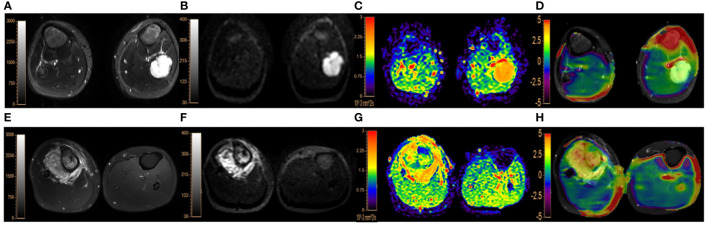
(A–D). A 9-year-old male patient with endochondroma in the left fibula: (A), fat-suppressed T_2_WI showing a irregular hyperintense lesion in the left fibula with marked dilatation, lobulated changes and intact bone cortex; (B, C), DWI image (b=1000 s/mm^2^) and ADC map, both showing the lesion with slightly uneven high signal intensities, with the lesion ADC value of 1.99×10^-3^ mm^2^/s; (D), APTWI fused on fat-suppresed T_2_WI with the lesion showing the MTRasym(3.5 ppm) value of 1.73%. (E–H). a 17-year-old male with osteosarcoma in the right proximal tibia accompanied by surrounding soft tissue mass: (E), fat-suppressed T_2_WI showing mixed high signal and irregular bone destruction in the right proximal tibia with surrounding soft tissue mass; (F, G), DWI image (b=1000 s/mm^2^) showing obvious hyperintensity and ADC map showing hypointensity with the lesion ADC value of 1.18×10^-3^ mm^2^/s; (H), the APTWI image fused on fat-suppresed T_2_WI with the lesion MTRasym(3.5 ppm) value of 4.07%.
